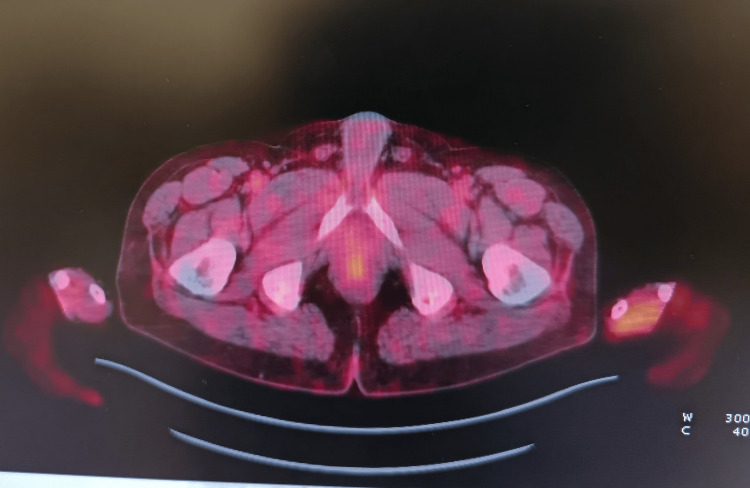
No distant metastases was noted. Mild postoperative FDG uptake in the inguinal region (SUVmax = 2.9), consistent with inflammatory change was seen. FGD: fludeoxyglucose; SUVmax: maximum standard unit value
